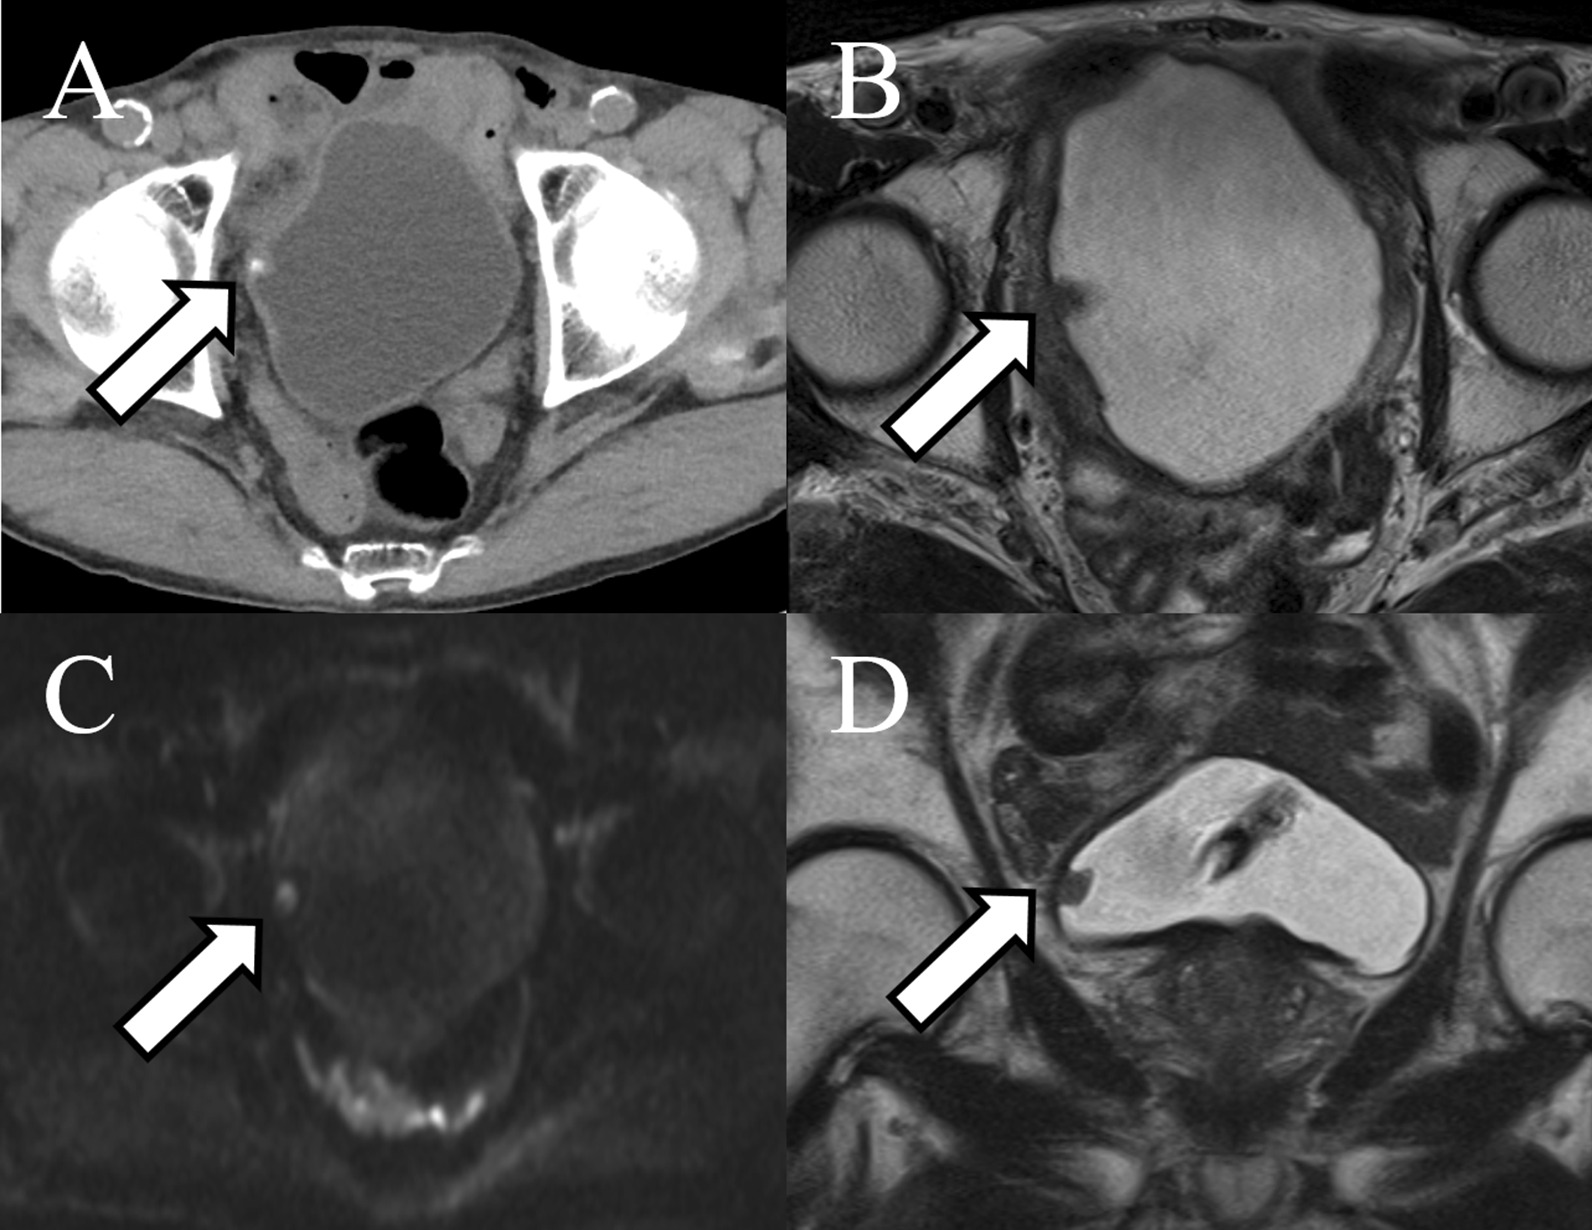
A Abdominal computed tomography showing a mass with calcification on the right wall. Magnetic resonance imaging also showed the tumor. B Axial section of a T2-weighted image. C The tumor showed a high signal intensity on diffusion-weighted imaging. D Coronal section of a T2-weighted image. The arrow points to the tumor
